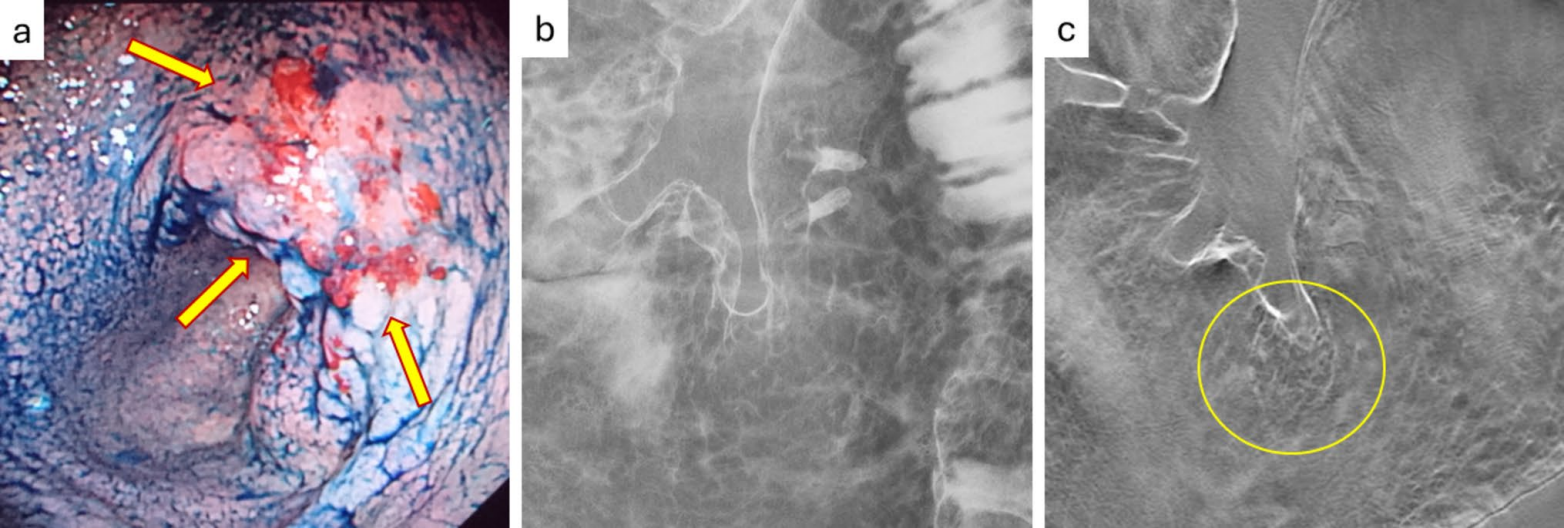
A case of depiction of early gastric cancer 1 (80 yr, male, type IIb + IIc). This figure shows an example of early gastric cancer visualized using different diagnostic methods, where endoscopy a clearly depicts the lesion, conventional Upper Gastrointestinal (UGI) radiography b fails to show it, highlighting the limitations of using only UGI for detection, and tomosynthesis c provides a layered image of the mucosal surface, making it clearly easier to identify the lesion. This comparison demonstrates that combining UGI and tomosynthesis can improve the visibility and detection accuracy of early gastric cancer
